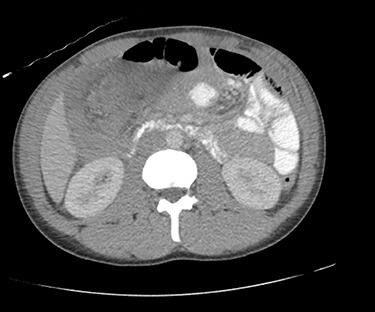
CT of the abdomen and pelvis with axial view showing ileo-colic intussusception.
